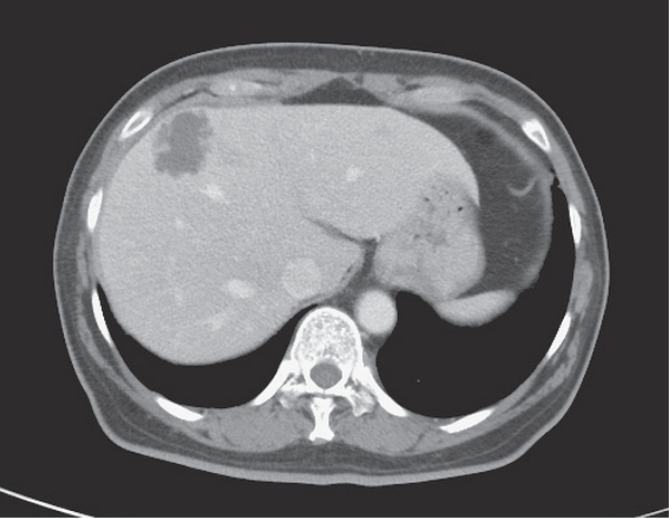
Abdominal CT scan showing a liver abscess.
